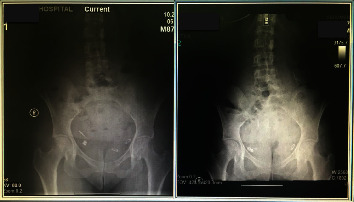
Two abdominal X-ray images of the patient. On the right, abdominal X-ray on first day of foreign body ingestion. On the left, 10 days after foreign body ingestion. Both images shows a right lower quadrant pointed metal object.
